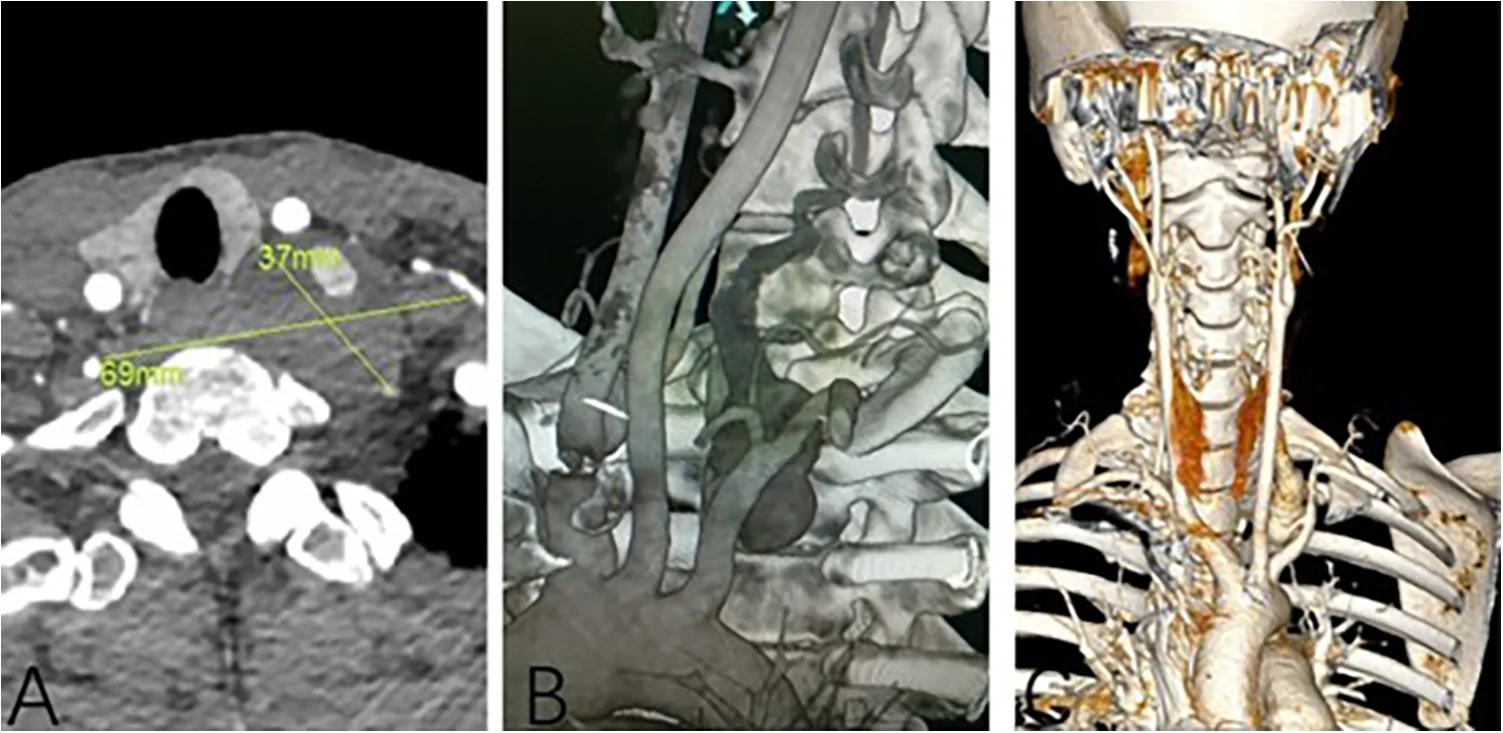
Contrast-enhanced CT (A) and 3D-CTA (B,C) images showing a thrombosed aneurysm in the V1 segment of left vertebral artery. The cross section shows a 69 × 37 mm thrombosed aneurysm in the left lower neck. CT, computed tomography; 3D-CTA, three-dimensional computed tomography angiography.
